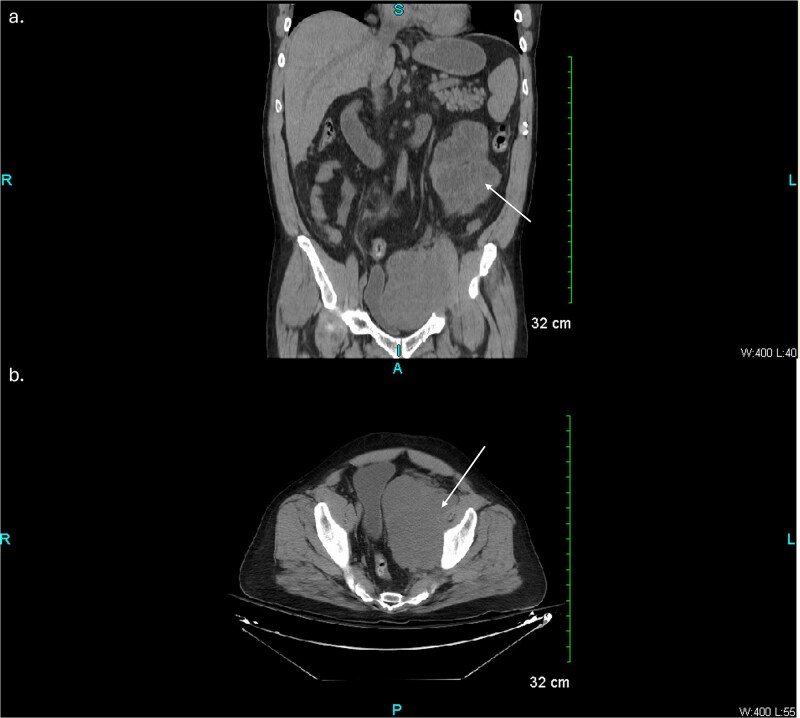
Pre-operative abdominal/pelvic CT scan without contrast (coronal and axial views).
